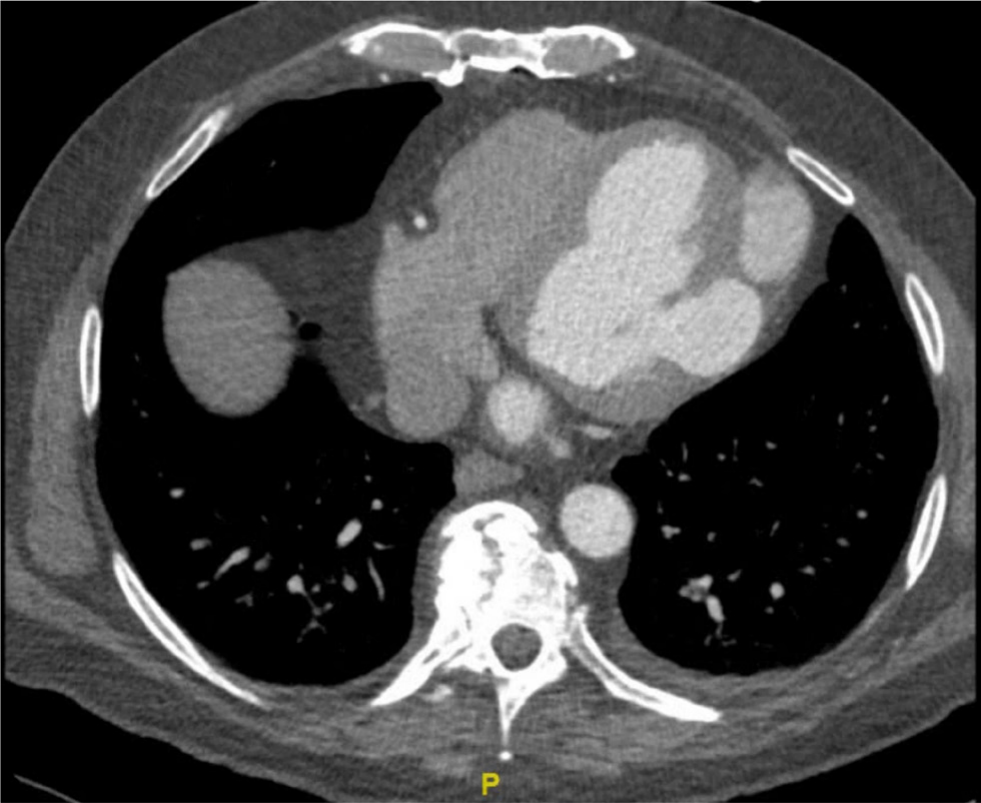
Computed tomography showing a large bilobed posterolateral left ventricular pseudoaneurysm.
